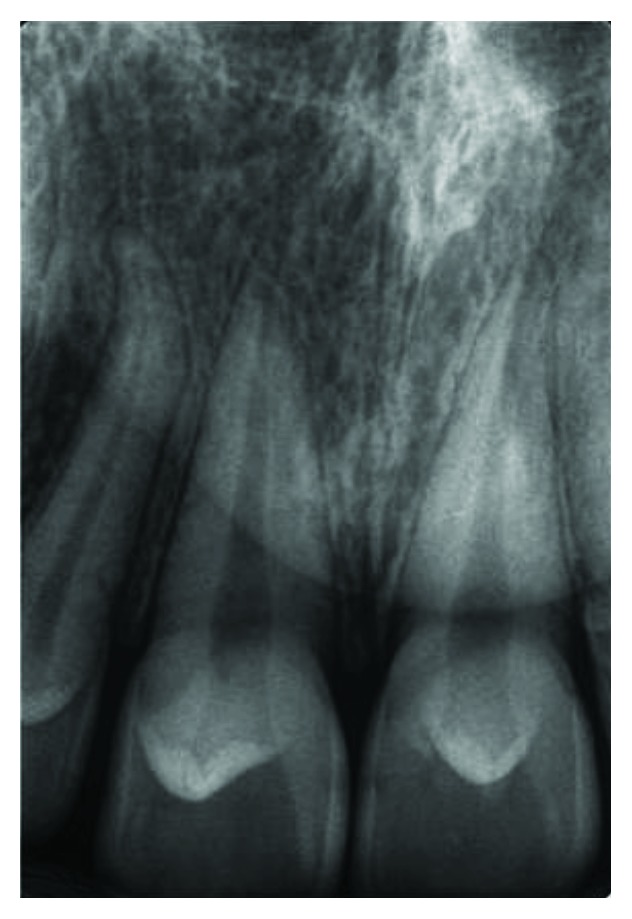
Case 4: periapical view for central incisors.
